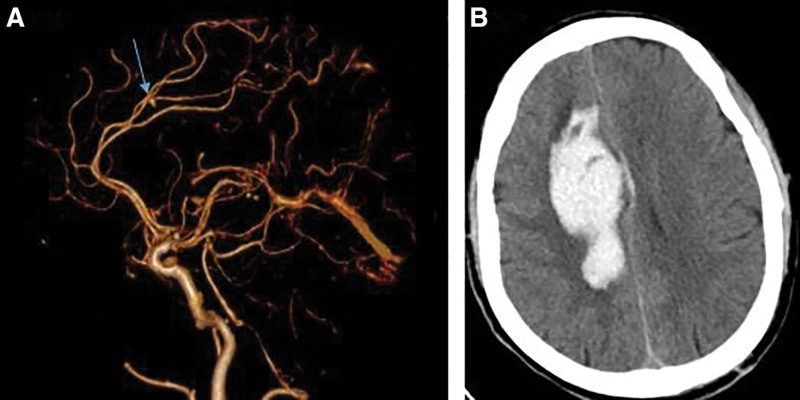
(A) CTA showed right anterior cerebral artery A4 segment aneurysm, about 0.3 cm × 0.3 cm × 0.4 cm. (B) Bilateral frontal and temporal lobe brain contusion; cerebral hemorrhage in the right corpus callosum and cingulate gyrus, left temporal parietal bone linear fracture with left temporal subdural hematoma; subarachnoid hemorrhage. CTA = computed tomographic angiography.
